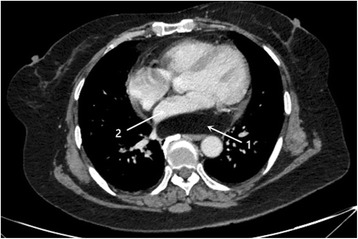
CTA showed that a large low density mass located on the posterior wall of the left atrium. The left atrium was compressed. The tumor was not enhanced on CTA (1. Tumor,2. Left atrium)
